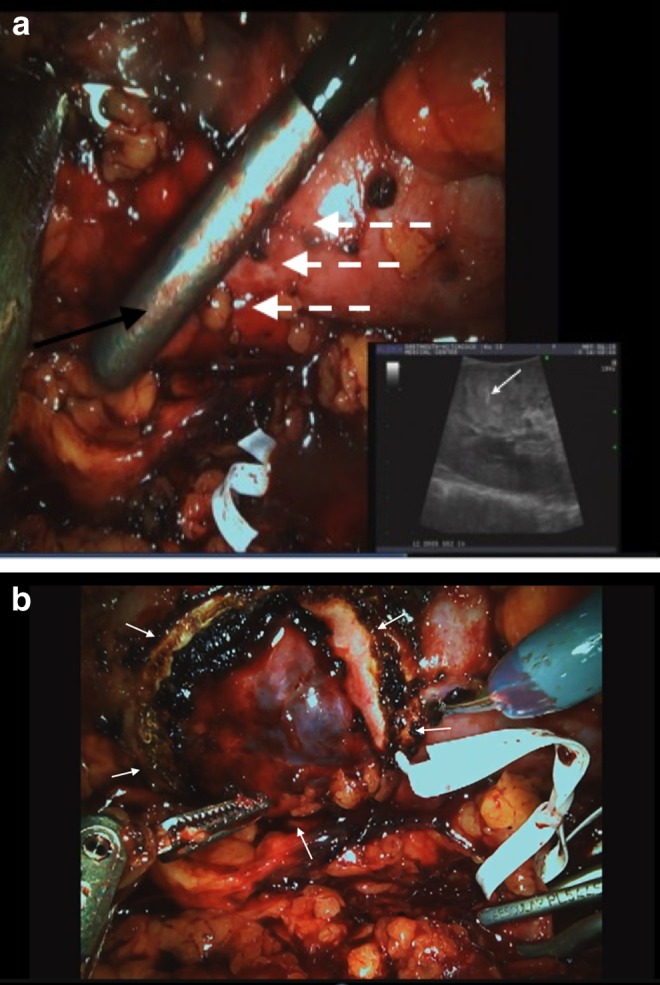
(a) Intracorporeal laparoscopic ultrasound demonstrating metal coil within indistinct, endophytic, isoechoic renal mass. *Dashed arrows*: area of suspected endophytic mass along medial aspect of the upper pole; *Black arrow*: ultrasound probe; *White arrow*: hyperechoic changes and artifact of metallic coil on ultrasound. (b) Capsular marking of anticipated tumor excision borders, marked with cautery using laparoscopic ultrasound as a guide. *Arrows* outline marking.
